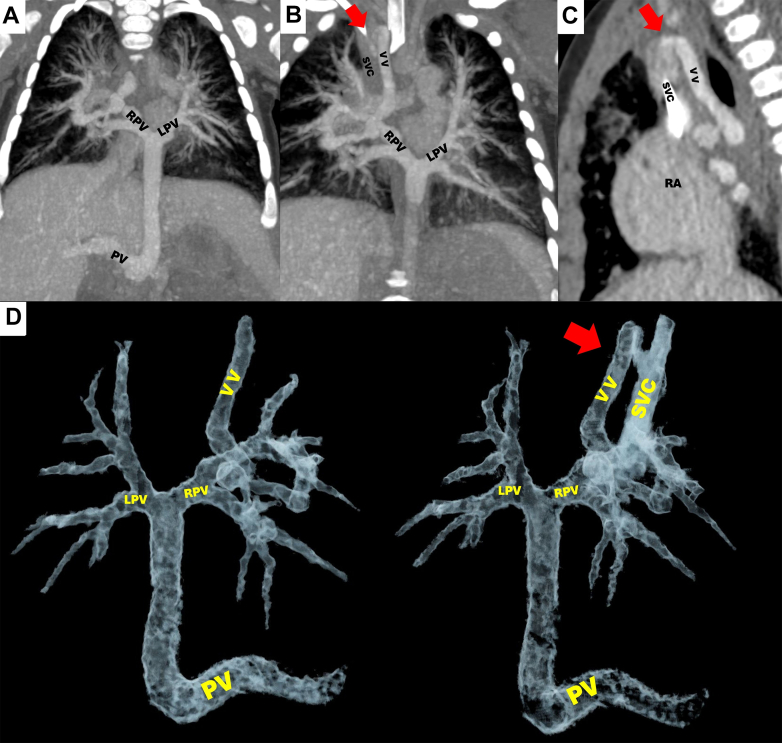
Patient 1 With Dual Drainage Total Anomalous Pulmonary Venous Connection Cardiac computed tomography angiography showing drainage of the right and left pulmonary veins to a common collector, which flows to the portal vein and SVC via a VV (red arrow). The VV receives segmental branches from the right upper and middle pulmonary lobes. (A, B) Coronal views and (C) sagittal view using maximum intensity projection. (D) Volume rendering technique 3-dimensional reconstruction imaging in posterior view. LPV = left pulmonary vein; PV = portal vein; RA = right atrium; RPV = right pulmonary vein; SVC = superior vena cava; VV = vertical vein.
